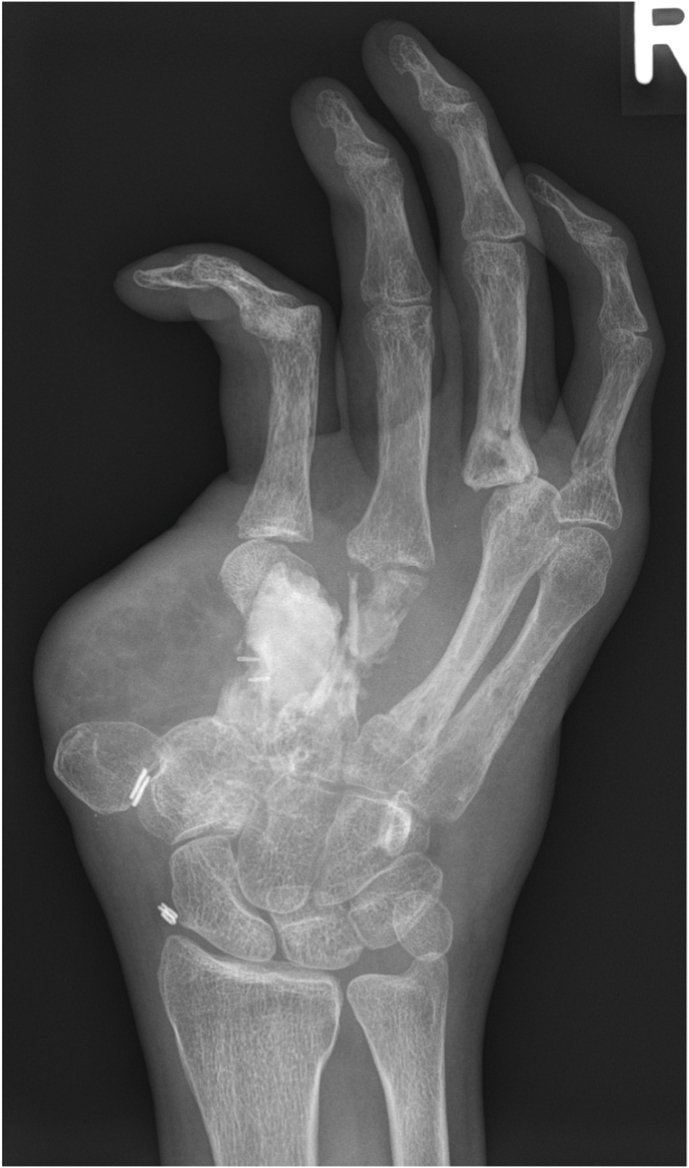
Pre-reconstruction radiograph 1.5 years after severe, devascularizing trauma to the right hand and multiple previous operations, resulting in osteonecrosis and collapse of the second and third metacarpal rays, despite the attempt to maintain length by using a bone cement spacer.
